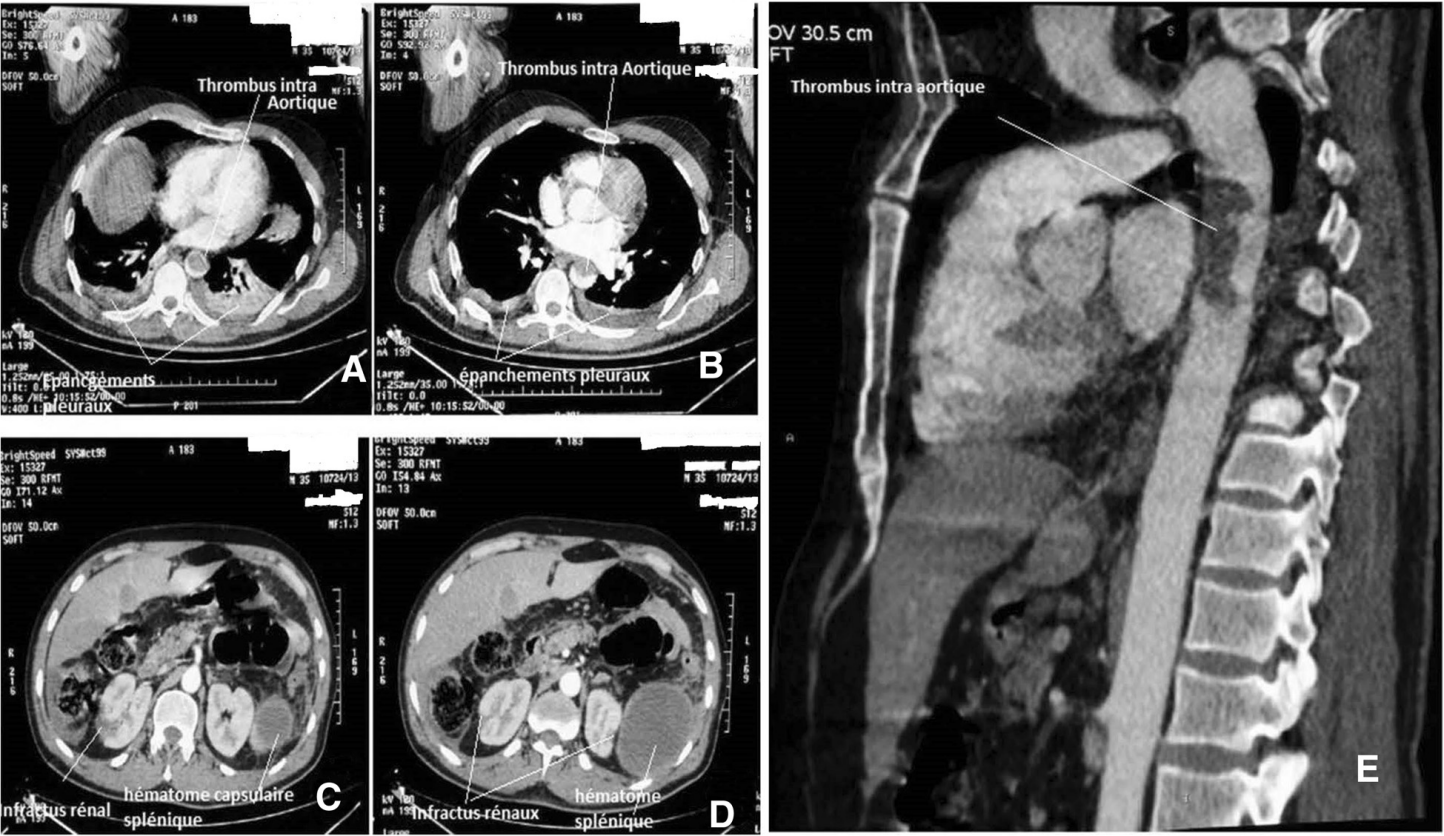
CT angiography showing the thoracic aorta of normal caliber without parietal atherosclerotic lesion, with an intra-aortic thrombus. Axial section; A, B. Sagittal reconstruction; E. Kidney infarct and peri-splenic hematoma, axial section C, D.
